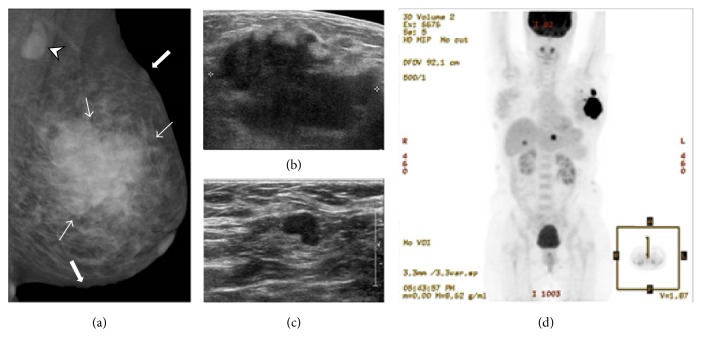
Left mediolateral oblique mammography shows an irregular hyperdense mass with indistinct margin (thin arrow) and axillary lymphadenopathy (arrowhead). Additionally, skin undulation is seen, indicating presence of cutaneous neurofibromas (thick arrow). Ultrasonography shows a large, hypoechoic, irregular mass with angular margin in the left breast (b) and multiple metastatic lymph nodes in the left axilla (c). PET-CT shows hepatic metastasis (d).
